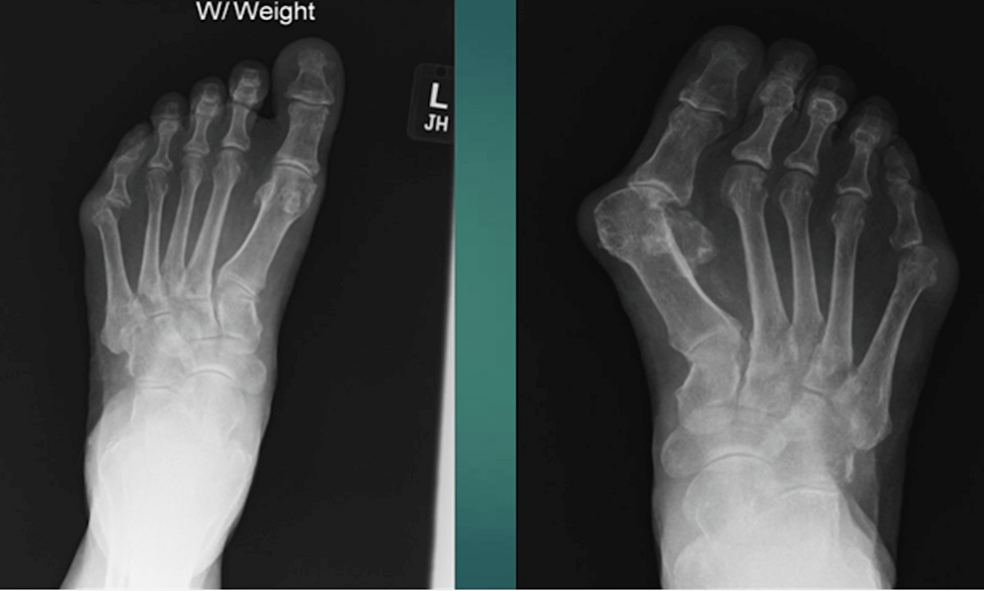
X-rays of feet revealing erosions of bilateral fifth metatarsophalangeal joints.
